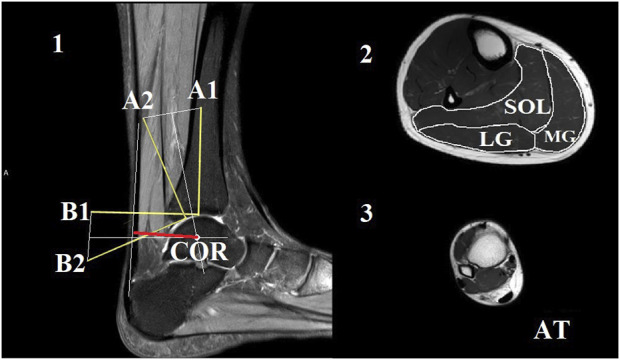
The Reuleaux graphical method was used to estimate the location of the center of rotation (COR) at the neutral joint position [1]. In this method, the talus was considered a fixed location, and the plantarflexion of the ankle was represented by the movement of the tibia with markers A1 and B1 to the next joint position marked with A2 and B2, respectively. Perpendicular lines were drawn to the bisectors of the connecting lines of A1-A2 and B1-B2. The intersection of these perpendicular lines marked the COR. The line of action for the Achilles tendon was marked in the image (indicated by the orange line). The length of the perpendicular line between the COR and the action line of the Achilles tendon (indicated by the red line) was considered as the length of the Achilles tendon moment arm. Representative magnetic resonance images from the middle of the lower leg were used for the calculation of cross-sectional areas [2]. The soleus (SOL), medial gastrocnemius (MG), and lateral gastrocnemius (LG) compartments were outlined manually. Additionally, a sample image of the maximal distal Achilles tendon (AT) was included [3].
